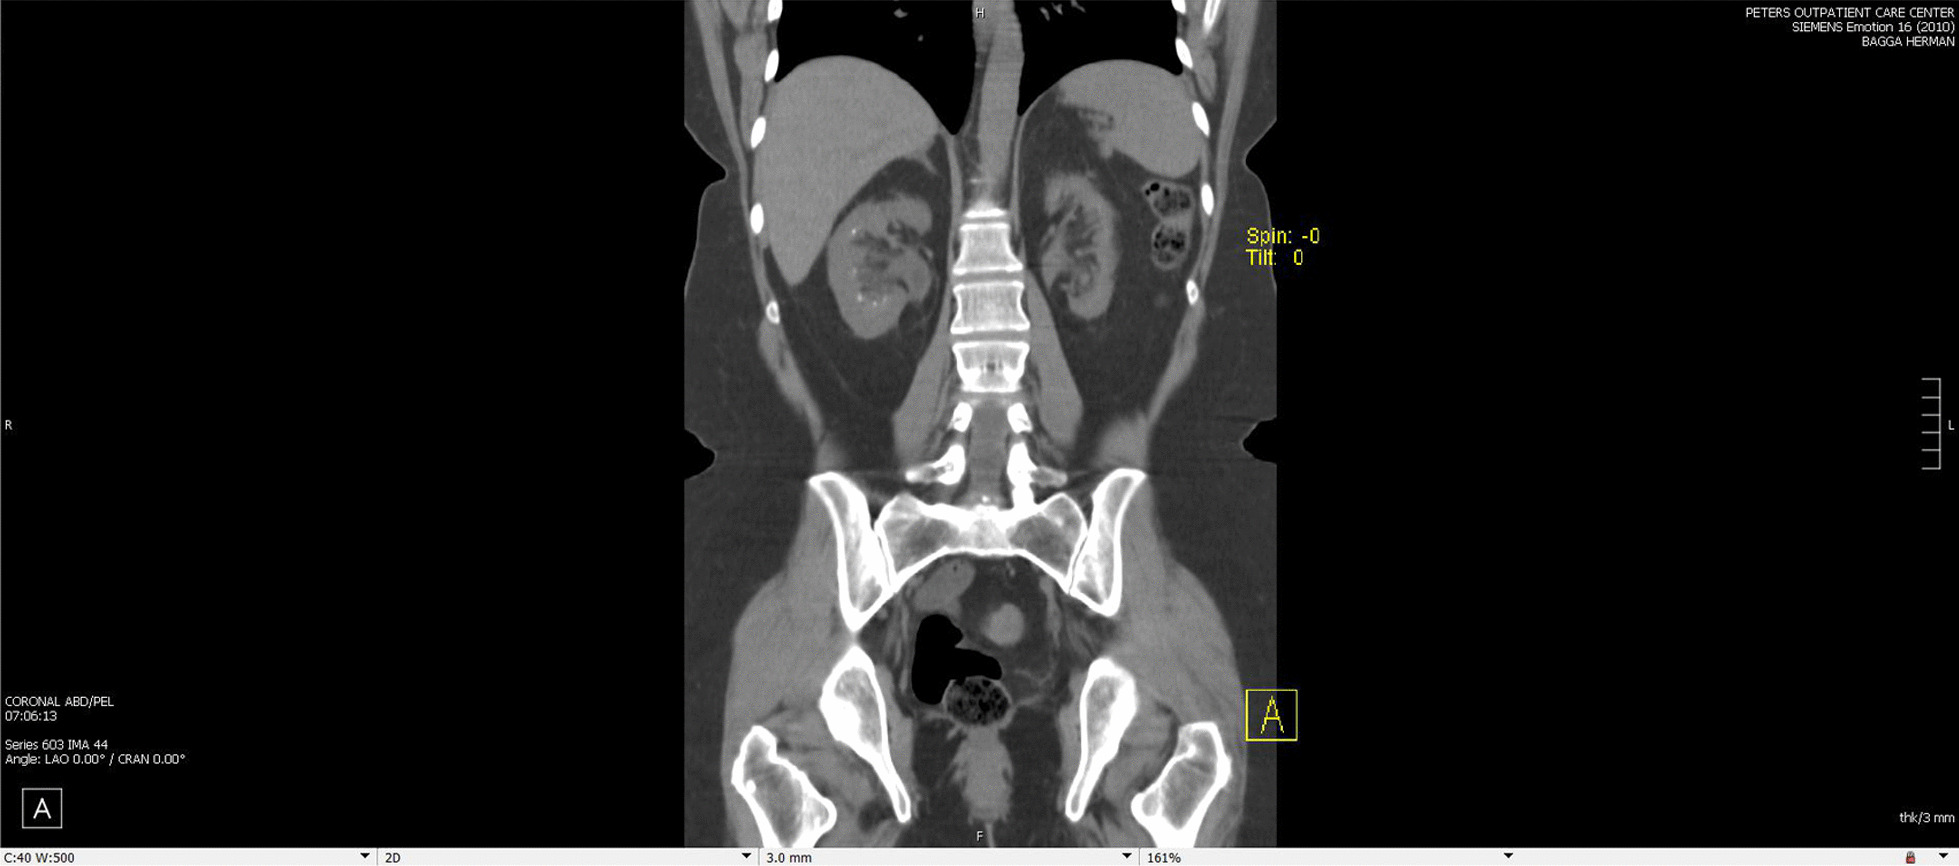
CT scan of patient from 2021 noting persistent nephrocalcinosis but no large stones and progressive left atrophy
